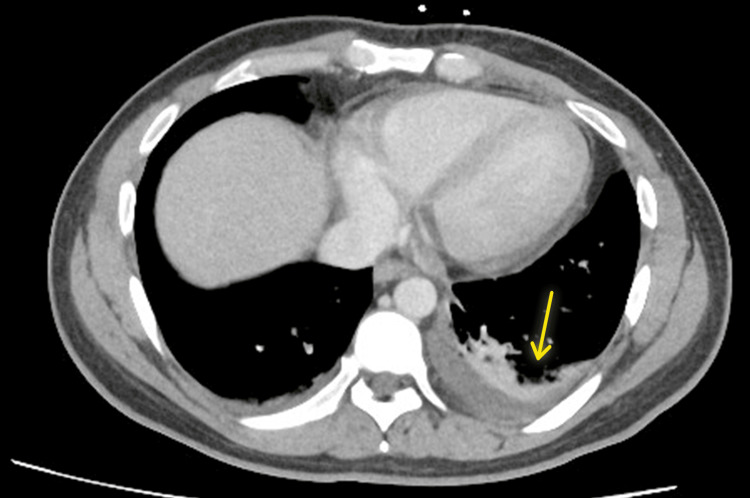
Computed tomography of the chest (axial cut) demonstrating a small left-sided pleural effusion with underlying atelectasis (yellow arrow). Yellow arrow showing the pleural effusion and atelectasis.
